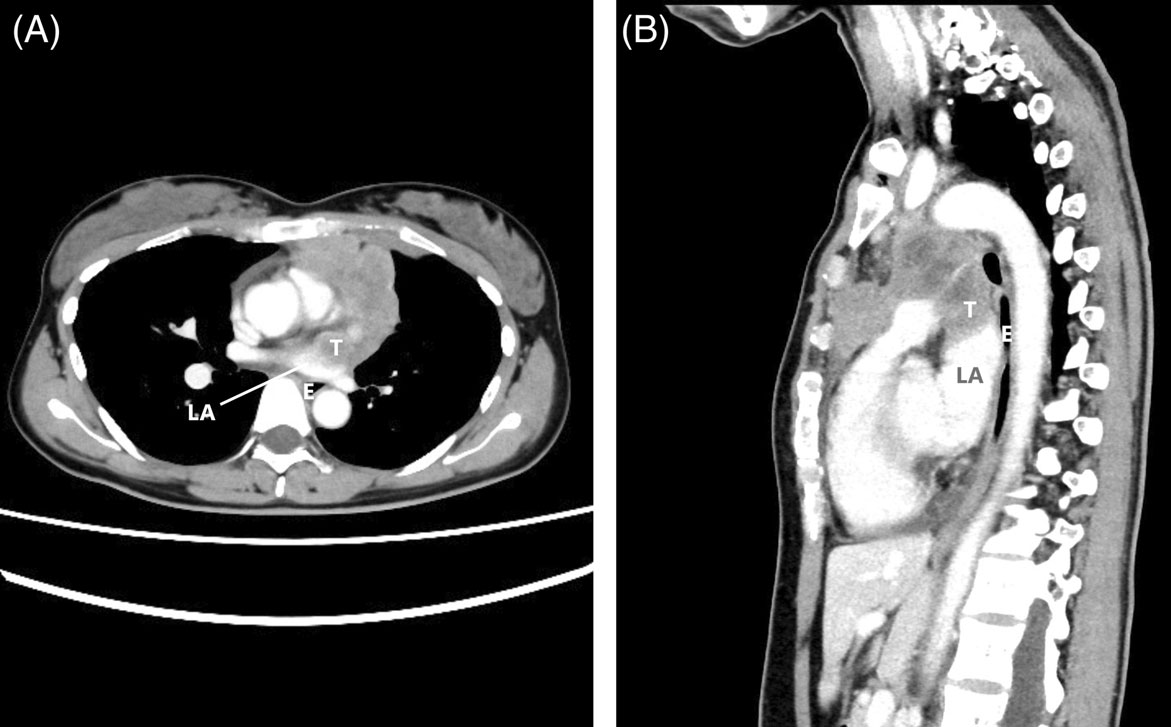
Enhanced computed tomography findings. Chest CT reveals suspected partial invasion of tumour adjacent to the heart into the left atrium. CT, computed tomography; LA, left atrium; T, tumour; E, oesophagus
